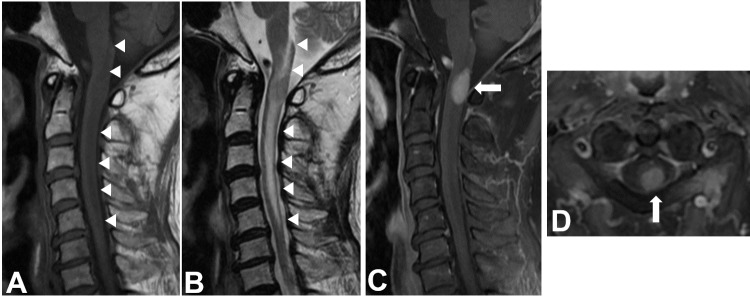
Preoperative magnetic resonance imaging Sagittal magnetic resonance imaging (MRI) showing an intramedullary mass in the cervical cord at the level of C1 associated with syringomyelia expanding from the medulla to the level of C5 (arrowhead). The lesion appears as an isointense mass on the T1 and T2-weighted images (A and B) and as a homogeneously-enhanced mass with gadolinium (C) (arrow). Axial MRI showing a mass lesion largely displacing from the spinal cord (D) (arrow).
